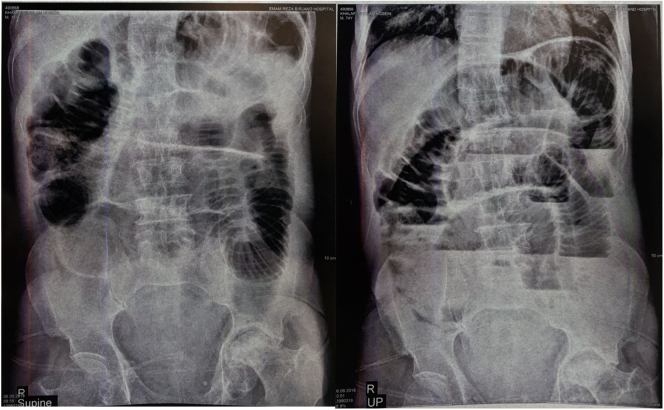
Supine (AP) and upright (PA) radiographs of the abdomen showing visible dilatation and multiple fluid levels in the intestine, suggesting obstruction.
